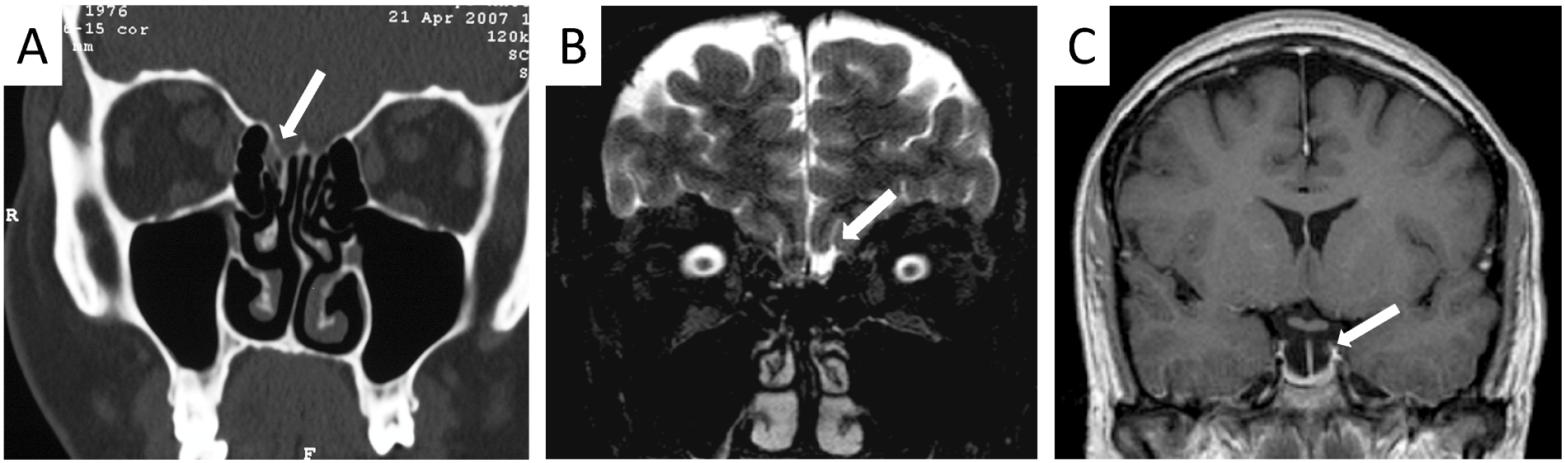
A) CT: CSF-leak of the lamina cribrosa right side. B) MRI: CSF-leak of the lamina cribrosa left side. C) MRI: empty sella syndrome.
